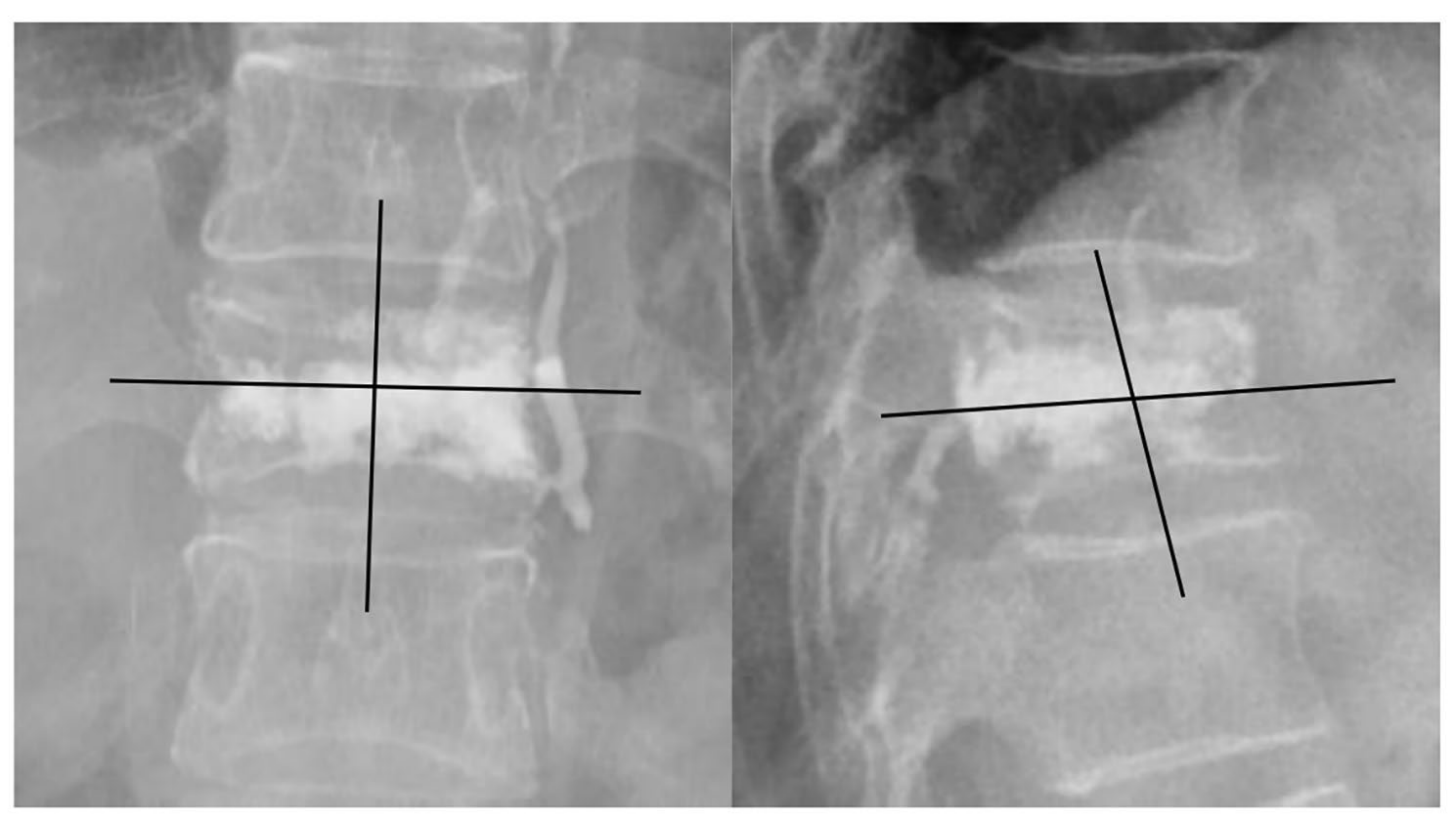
The vertebra was divided into 4 quadrants on lateral and anteroposterior radiographs. Each quadrant filled more than 1/3 with bone cement was scored as 1 point, and the total score was the bone cement distribution score
